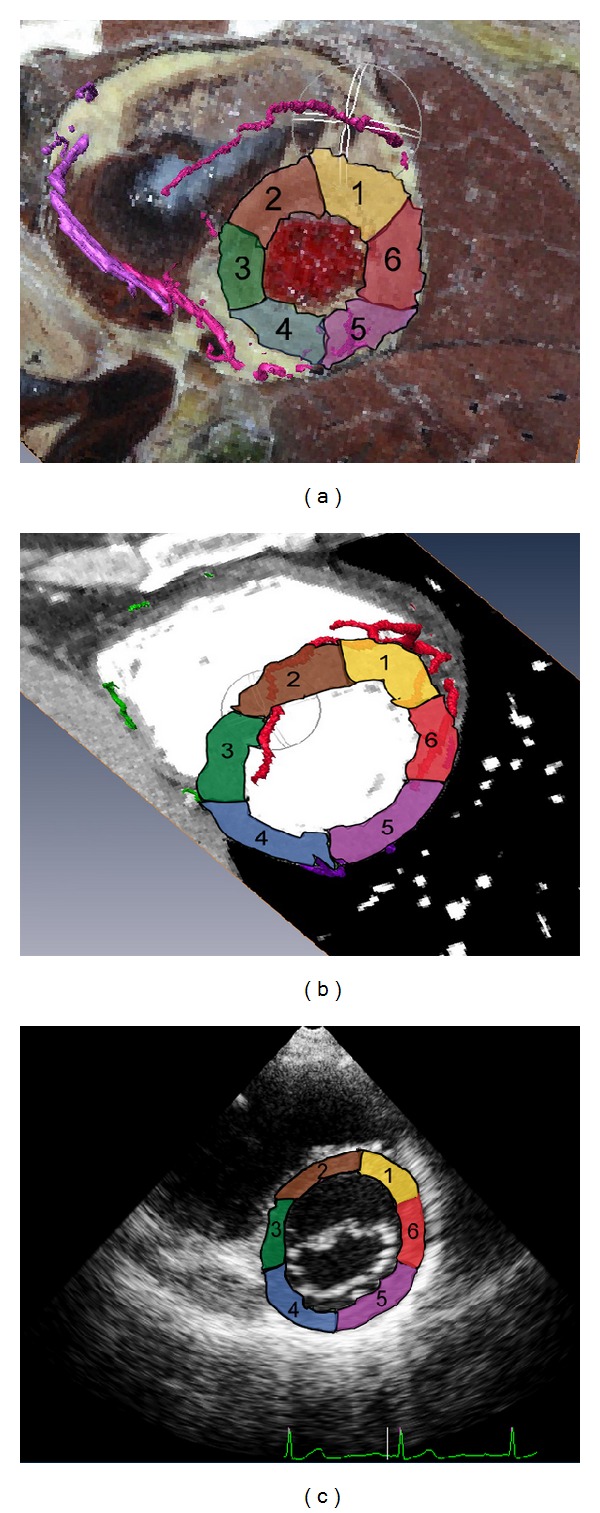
Short-axis sections of left ventricle at the level of the mitral leaflet. (a) Fusion display of the CVH dataset and the coronary artery model. (b) Fusion display of the cardiac CT dataset and the coronary artery model. (c) Short-axis section of the left ventricle at the level of the mitral leaflet revealed by echocardiography.
